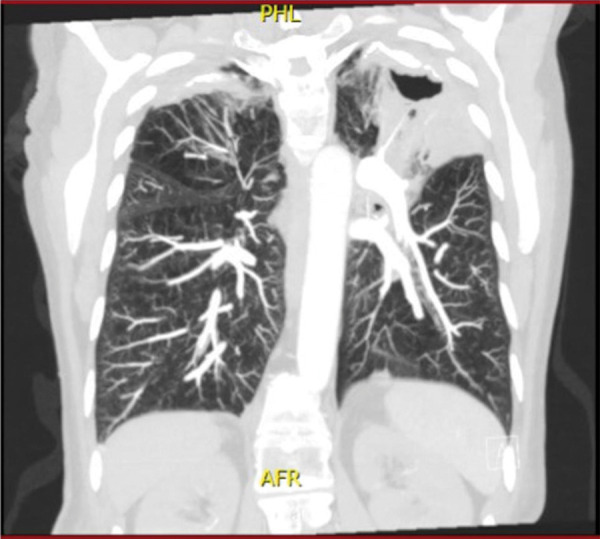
Computed tomography scan of the lungs revealing emphysema, bilateral infiltration, and calcification located in the upper lobes, and a cavity with no air-fluid level on the upper left lobe. There are no signs of pulmonary hemorrhage.
